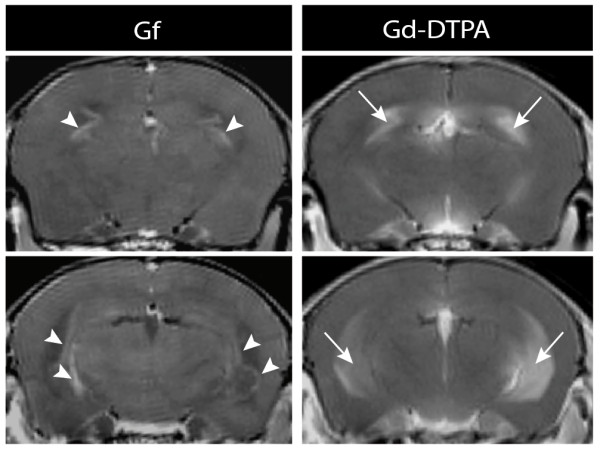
Early Gf enhancement of the choroid plexus. Coronal T1-weighted images are depicted 1 h after Gf injection (left column) and immediately after Gd-DTPA injection (right column). Applying Gf, distinct enhancement of the choroid plexus (arrow heads) and other CVO was evident early after intravenous injection and allowed for a reliable quantitative signal analysis. In contrast, after injection of Gd-DTPA, the disruption of the blood-CSF barrier frequently caused leakage of contrast agent into the CSF (arrows) obscuring the choroid plexus and periventricular lesions.
